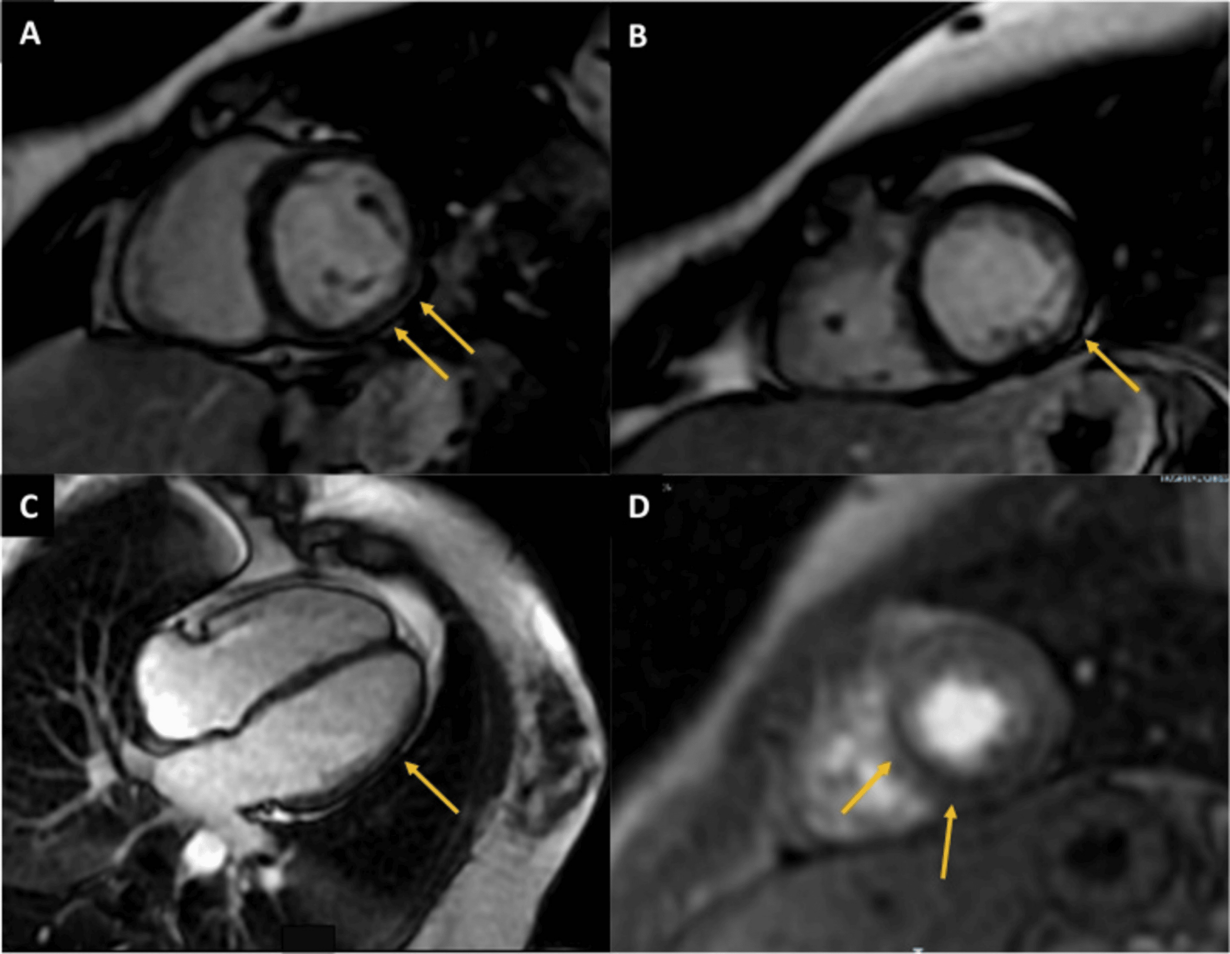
Cardiac magnetic resonance imaging performed on the patient Images A-C: Late gadolinium enhancement (LGE) sequences in short-axis (A and B) and four-chamber (C) views showing subepicardial enhancement in the posterior and inferior walls (yellow arrows), a pattern consistent with non-ischemic myocardial injury of probable inflammatory origin. Image D: First-pass perfusion sequence demonstrating a subendocardial perfusion defect in the septal and inferior walls of the mid-apical segment (yellow arrows), suggestive of ischemia without a clear coronary territory.
